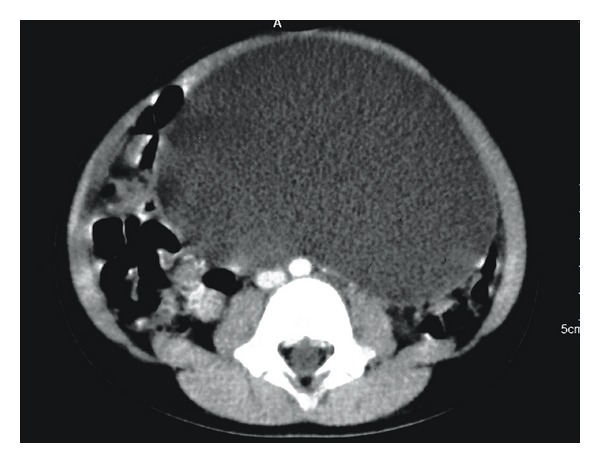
Abdominal CT showing a 13 cm × 8 cm × 12 cm cystic abdominal mass.
